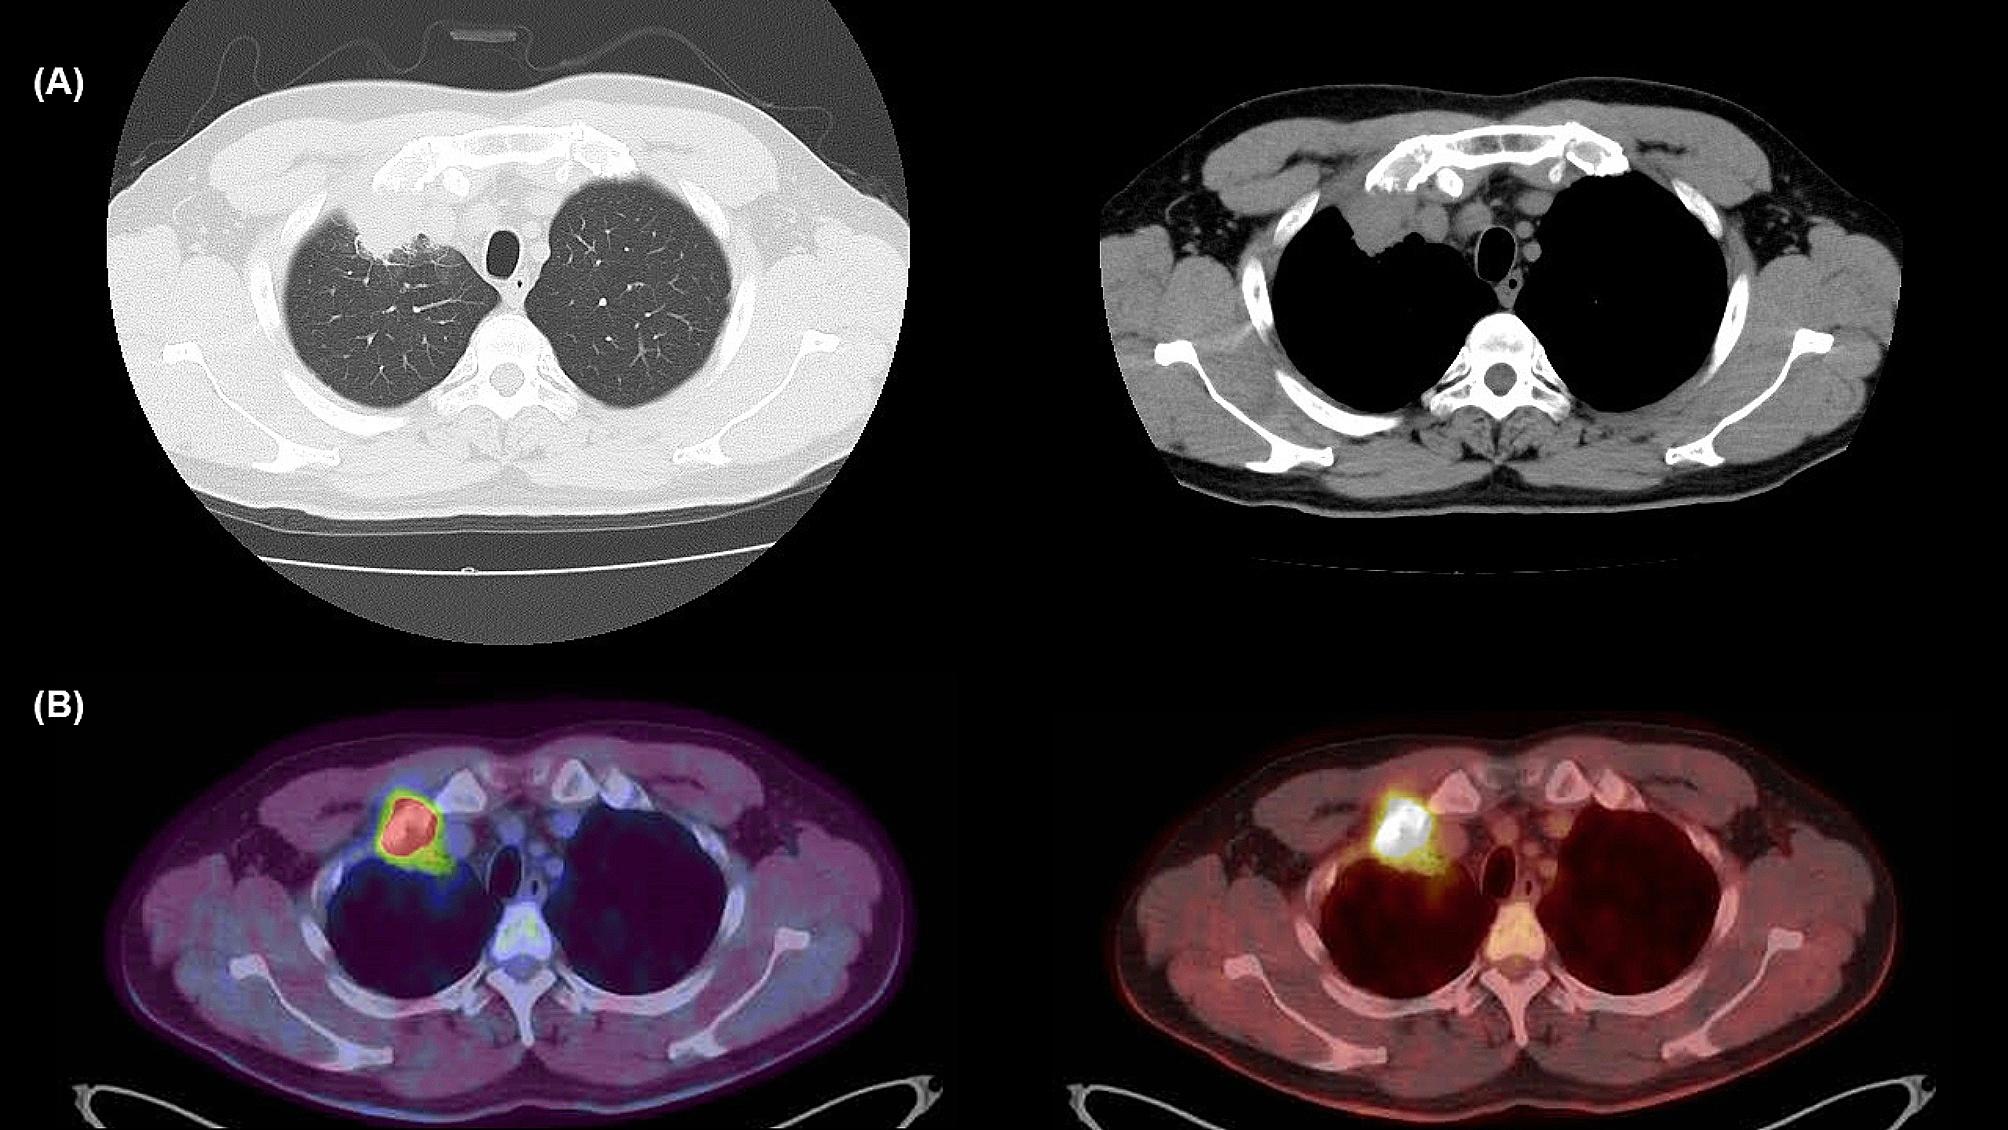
(A) Computed tomography on the 12th day post-admission shows a tumoral lesion suspicious of infiltration into the right first rib and intercostal muscles, with changes in the density of the lung parenchyma around the tumor. These findings are suggestive of extension of the lesion along the lymphatic vessels and stroma. (B) 8 F-Fluorodeoxyglucose positron emission tomography-computed tomography on the 17th day post-admission shows a tumoral lesion with a high degree of accumulation with a maximum standardized uptake value = 16.73
